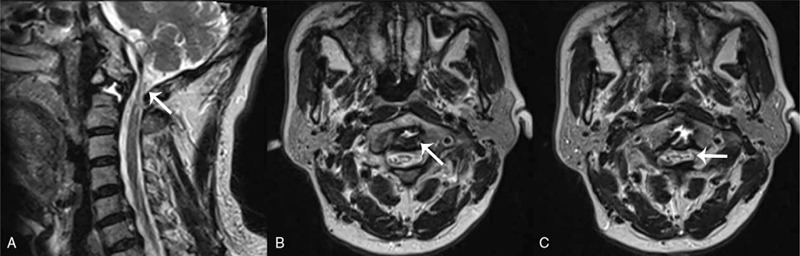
The T2-weighted phase of MRI. The sagittal image showed compression of the spinal cord at the C1-C2 segment and strip-like high signal in the spinal cord (A), and the axial images revealed severe cervical stenosis at the C1-C2 segment (B, C). MRI = magnetic resonance imaging.
